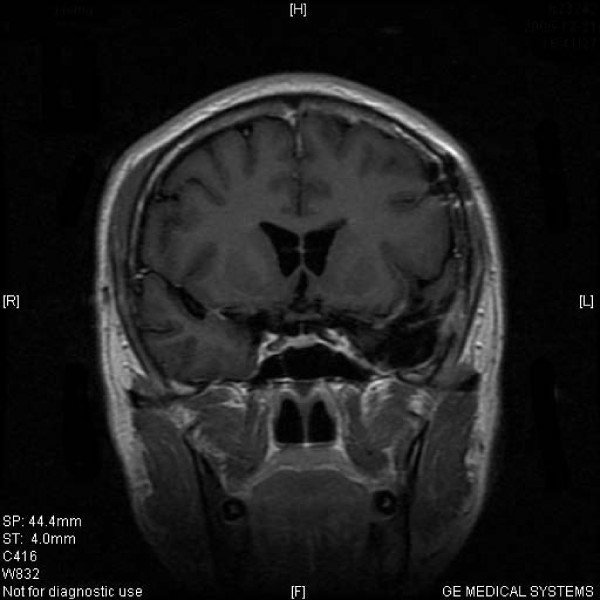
Typical MRI scan changes in ACTH adenoma. No enhancing mass lesion is seen in the sella turcia under the T1-weighted postcontrast MRI scan performed 2 years after GKRS. Patient 1's clinical symptom did improve. His serum ACTH level came down to 40.4 pg/ml, and his blood pressure was controlled within 140/80 mmHg.
